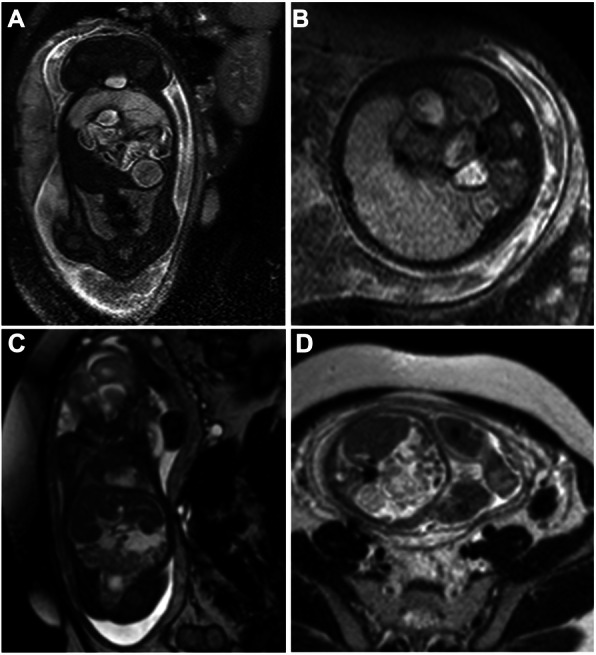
Fetal MRI findings of meconium peritonitis in two fetuses with existing cysts. a-b. A 34-week-old fetus received surgical intervention. A coronal T2-weighted image (a, SPAIR) reveals a cystic lesion in the left upper abdomen measuring 2.8 × 2.9 × 1.9 cm in size with a distinct thin border, linear-like incomplete separation and slightly high T2 signal inside. A transverse 1.5-T fetal T2-weighted image (b, TE = 15,000 ms, TE = 120 ms) shows that the bowel loops were pressed and shifted backward and dilated significantly with a diameter of 2.6 cm. c-d. A 27-week-old fetus received conservative treatment. A coronal T2-weighted image (c, SPAIR) shows a pseudocyst measuring 3.3 × 1.3 × 1.6 cm in size with irregular shape and high and slightly low T2 signal mixed inside. A transverse T2-weighted image (d, TR = 15,000 ms, TE = 120 ms) reveals a mild mount of ascites among bowel loops
